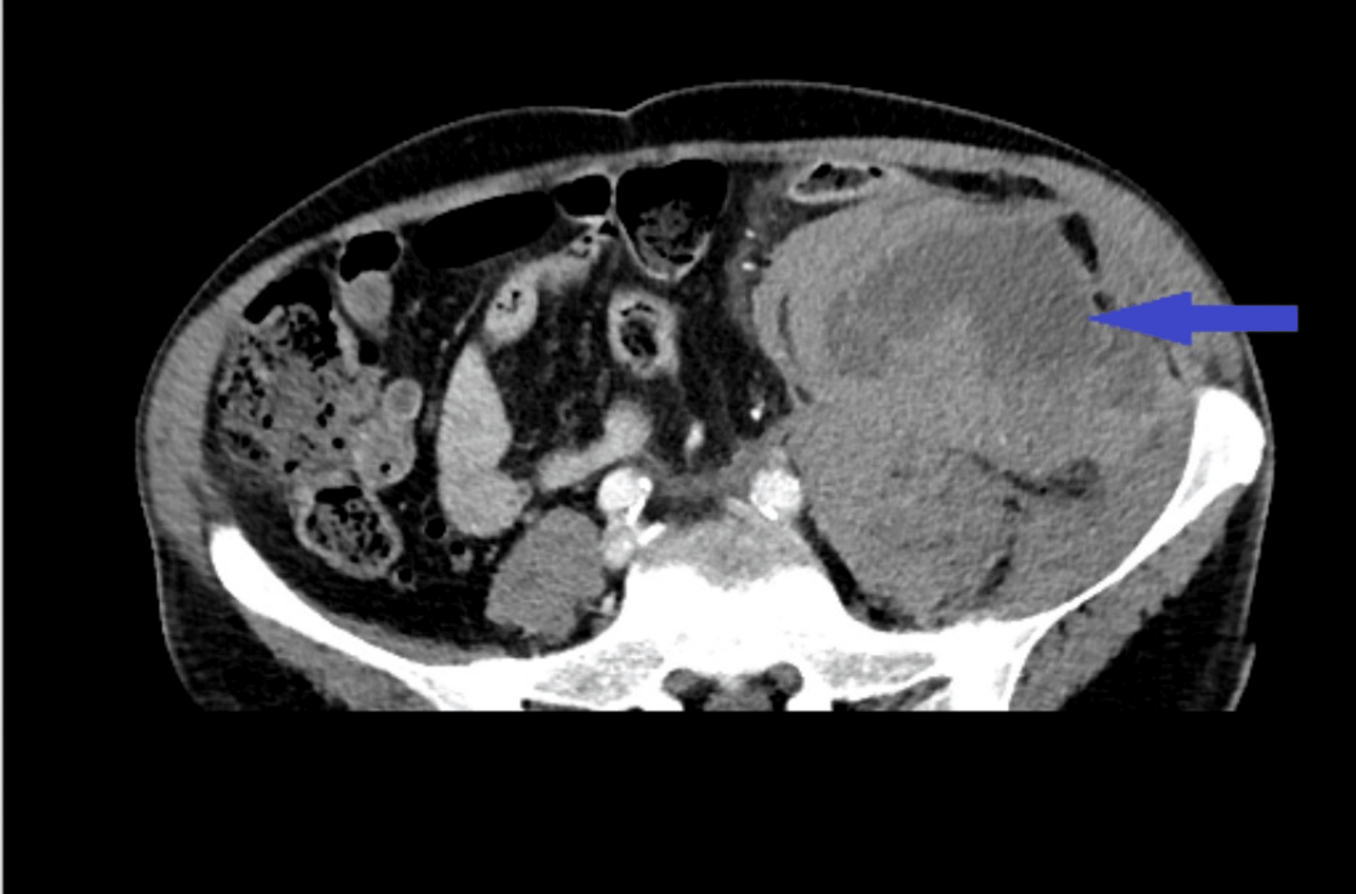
CT angiography of the patient revealing an actively bleeding large hematoma 25 x 9 cm in the retroperitoneal cavity (blue arrow).
